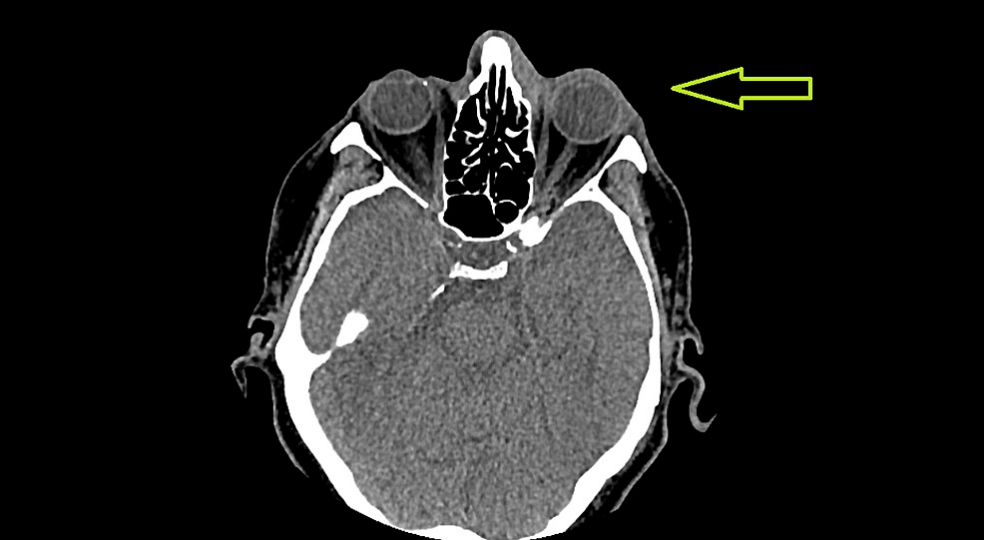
CT scan after radiotherapy to the affected area, showing marked improvement.
